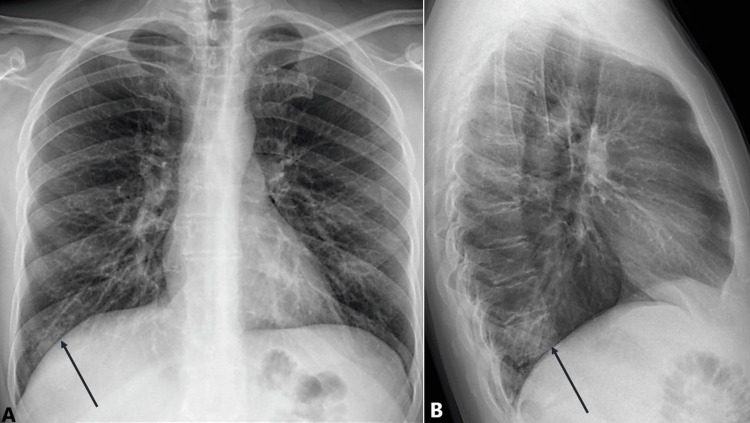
Chest X-ray at 3-week follow-up (A) Posterior-anterior view showing minimal residual findings (black arrow), reflecting near-complete resolution of the pneumonia and pleural effusion. (B) Lateral view confirming no significant abnormalities (black arrow), demonstrating the patient’s recovery.
